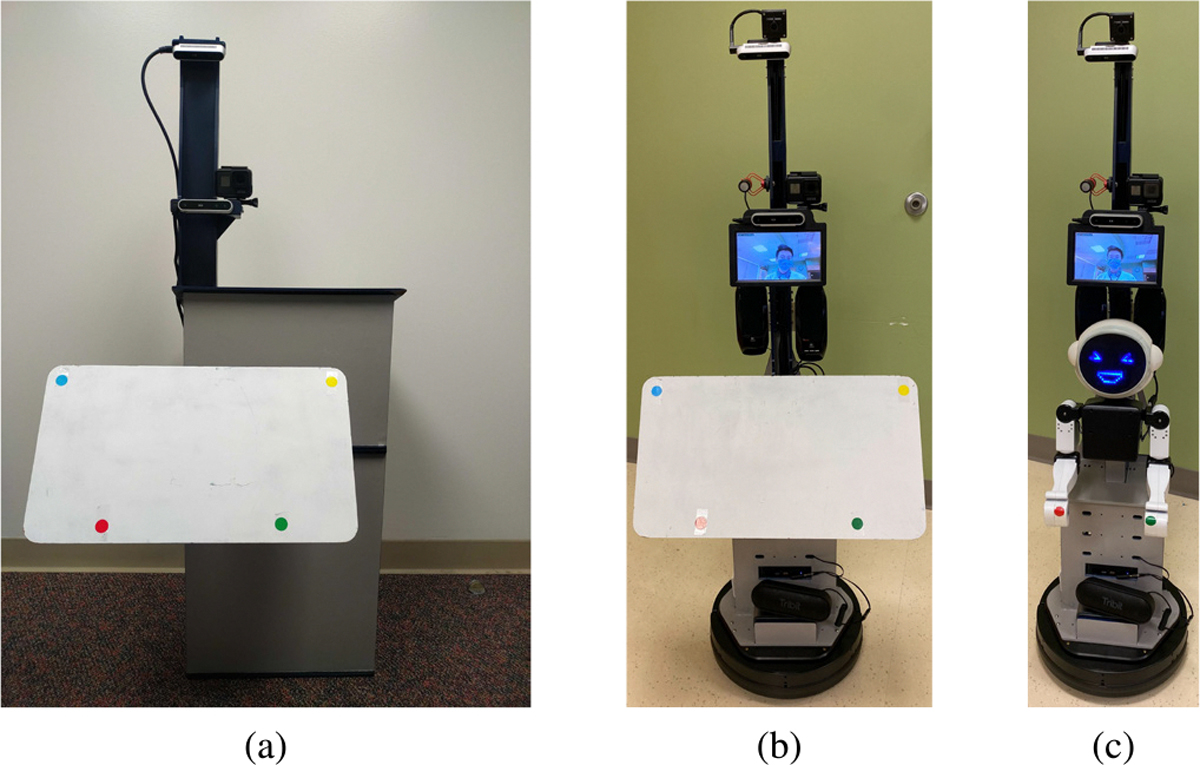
Different configurations for the Flo telepresence platform used throughout the study. (a) Face to face (FTF). (b) Classical telepresence (CT). (c) Social robot augmented telepresence (SRAT).
